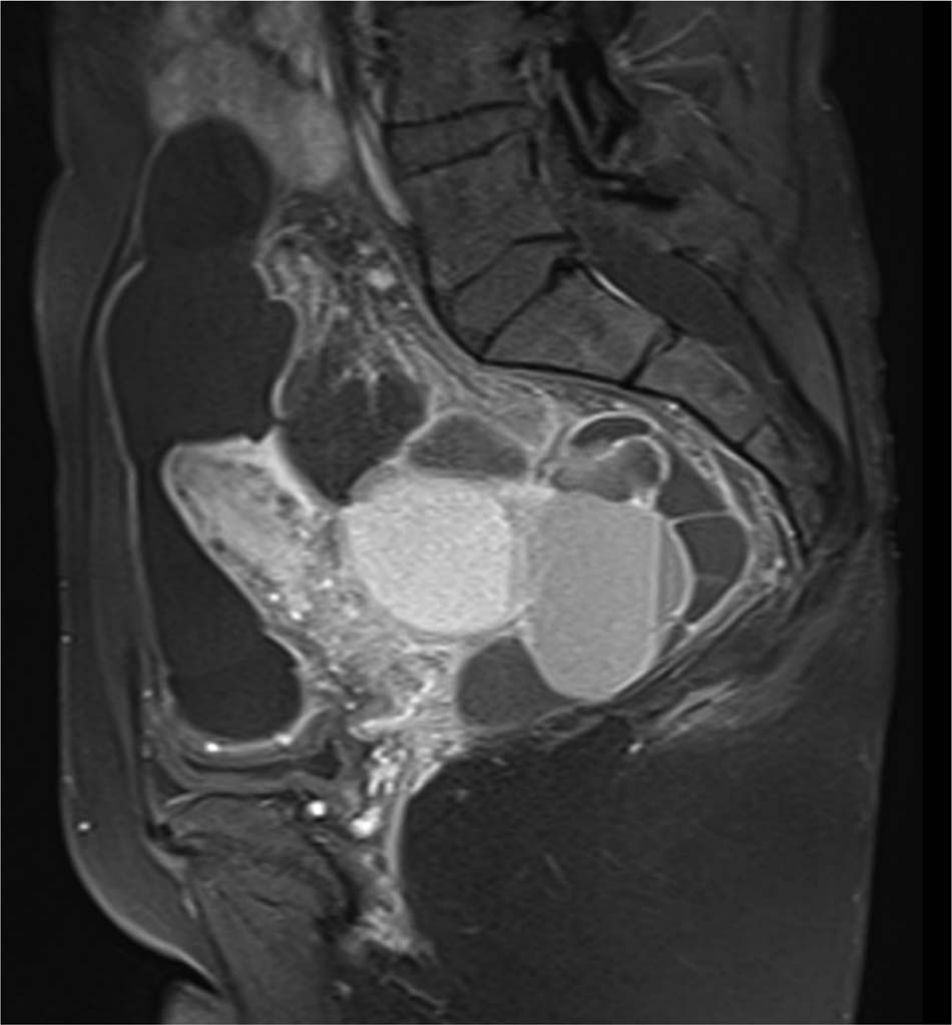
Sagittal T2-weighted pelvic MRI demonstrating a large multilocular cystic lesion occupying the pelvic cavity and displacing adjacent organs.
